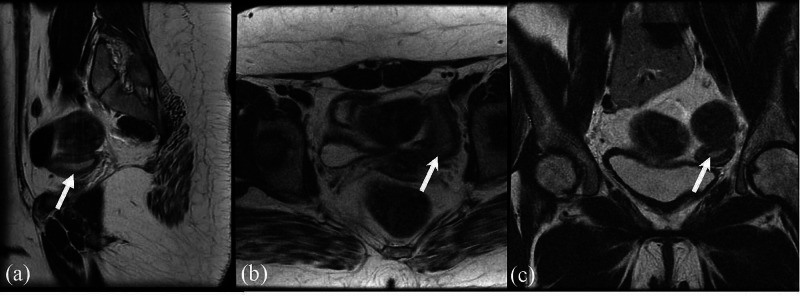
Repeated MRI of the uterus, after four years of management with OCP (a) sagittal T2 shows the uterus (arrow); (b) axial T2 shows the uterus (arrow); (c) coronal T2 shows the uterus (arrow) OCP: oral contraceptive pill
